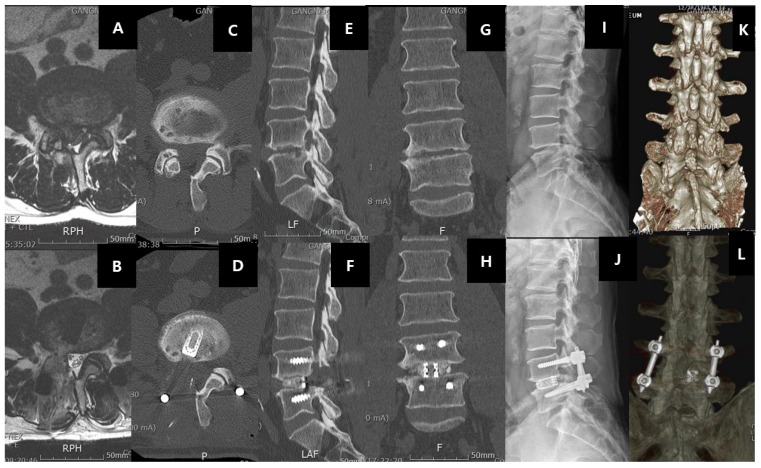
Right-sided L4/5 recurrent disc herniation after previous open laminotomy and discectomy 2 years ago, the patient underwent uniportal endoscopic transforaminal lumbar interbody fusion of right L4/5(ETLIF). A shows the recurrence of disc herniation with facet arthritis in the MRI axial cut of L4/5. B shows the same axial cut with left transforaminal lumbar interbody fusion showing resection of the facet joint and cage in an optimal position. C and D show the pre and post-operative status of the right facet. Note the previous right L4 laminotomy in C, the facet was resected, and the cage introduced in an optimal position as shown in D. E and F show the sagittal view of the pre and pos-operative status. Note the increase in the foraminal height and intervertebral height as a result of the right L4/5 ETLIF. G and H show the increase in the coronal disc height pre and post-operatively in right L4/5 ETLIF. I and J show the pre and post-operative standing neutral XR with the L4/5 interbody cage and standard posterior inserted pedicle screws in L4 and L5. K and L show the 3D reconstruction of pre and post-operative right L4/5 ETLIF.
